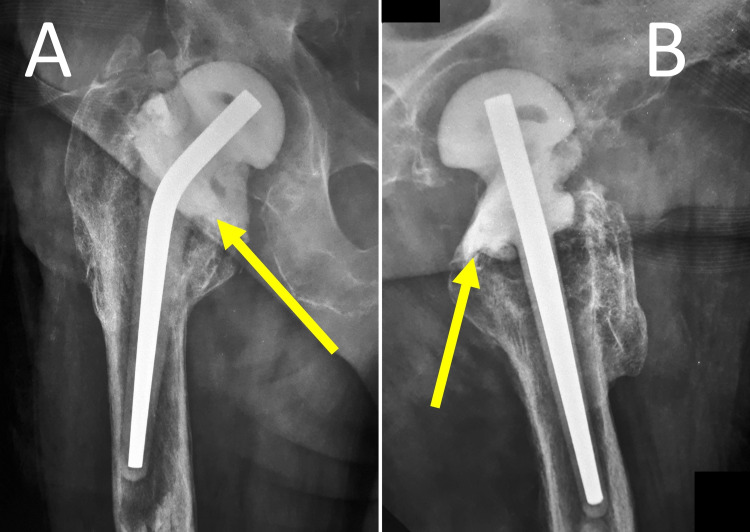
Two-stage revision of PJI after an infected hemiarthroplasty of the right hip using a prefabricated ALCS. Anteroposterior (A) and lateral (B) radiographs of the right hip. High-dose antibiotic-loaded cement (yellow arrows) has been added around the proximal part of the spacer to prevent complications such as migration, rotation and dislocation of the spacer or bone loss of the femur. PJI: Prosthetic joint infection, ALCS: Antibiotic-loaded cement spacer.
